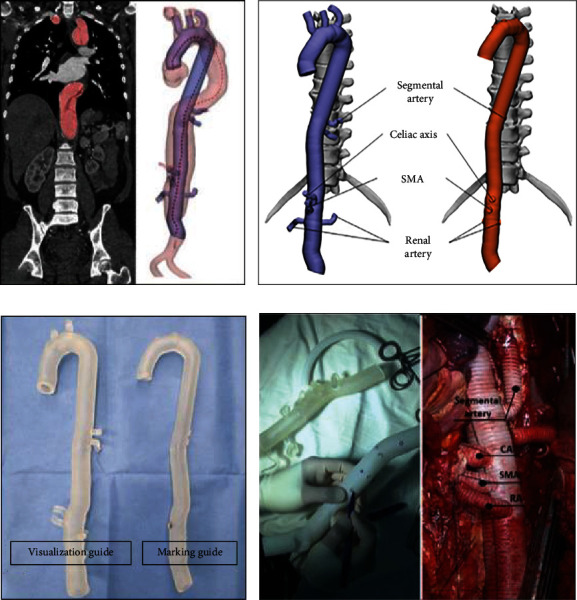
3D modeling processes for 3D aortic model. (a) Individual modeling of the central line of graft using the patient's computed tomography (CT) images. (b) 3D computerized models for the graft guides consisting of the visualization (left) and the marking (right). (c) 3D printed guides. (d) Intraoperative view of the 3D printed guides in the TAAA. SMA: superior mesenteric artery; CA: celiac artery; RA: renal artery; TAAA: thoracoabdominal aortic aneurysm [137]. Used with permission from Elsevier.
